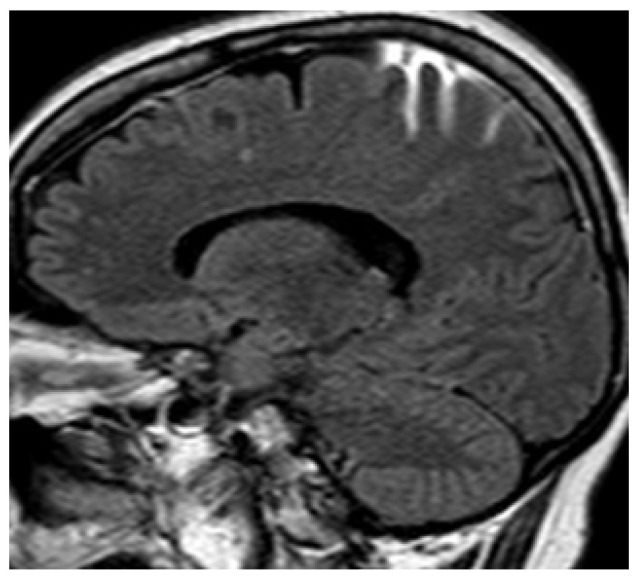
Sagittal fluid attenuated inversion recovery (FLAIR) image. Spontaneous subarachnoid hemorrhage in the precentral, central, and postcentral sulci in the context of cerebral venous thrombosis. Some cortical veins are visible, but their thrombosis cannot be verified because they are drowned in the spontaneous FLAIR signal of the subarachnoid hemorrhage.
